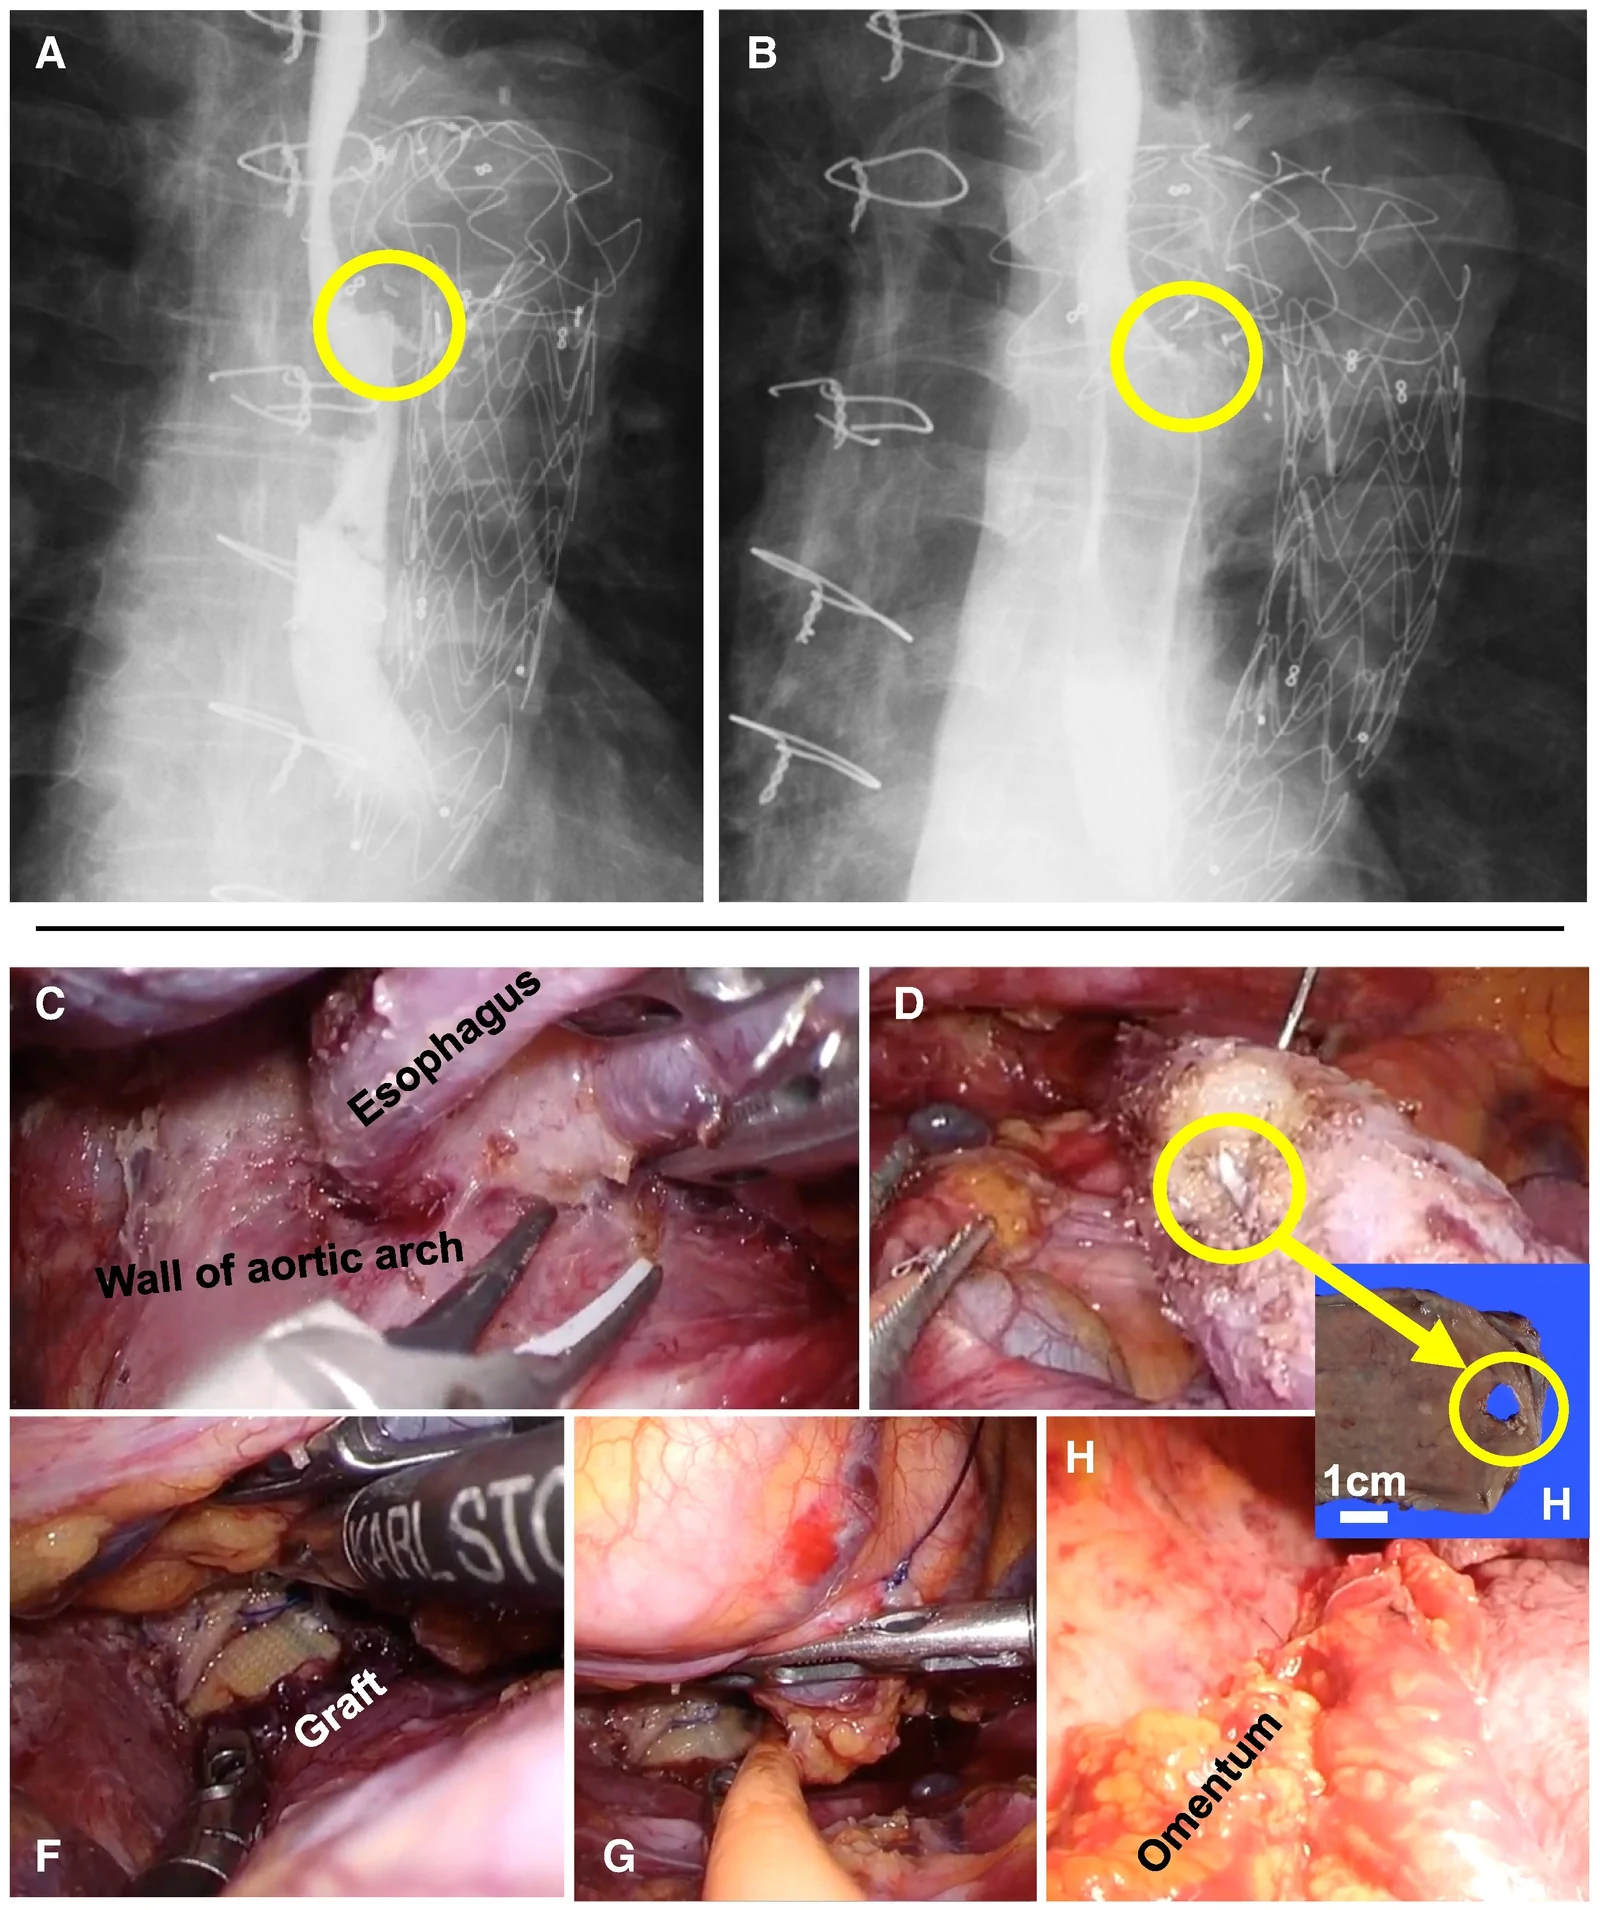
Computed tomography and aortography images. (*A* and *B*) Oesophagography demonstrating a persistent oesophageal perforation into the mediastinum (yellow circle). (*C*) Oesophagectomy was performed in a cranial-to-caudal direction, with careful dissection of the dense adhesions surrounding the aorto-oesophageal fistula. (*D*) The fistula between the oesophagus and pseudoaneurysm is exposed (yellow circle). (*E*) The diameter of the oesophageal perforation was ∼8 mm (yellow circle). (*F*) The artificial graft is shown. (*G*) After thorough debridement, the inflamed tissue was irrigated with saline. (*H*) The harvested omentum was placed around the artificial graft and within the mediastinum.
